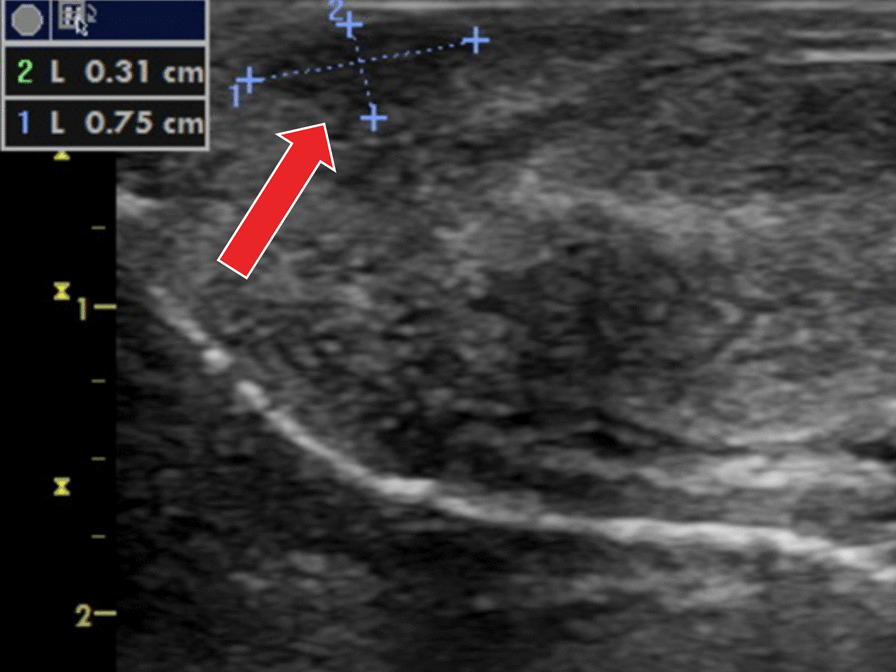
Ultrasound finding—a hypoechogenic, hypoperfused non-well defined area inside the glans (arrow)
